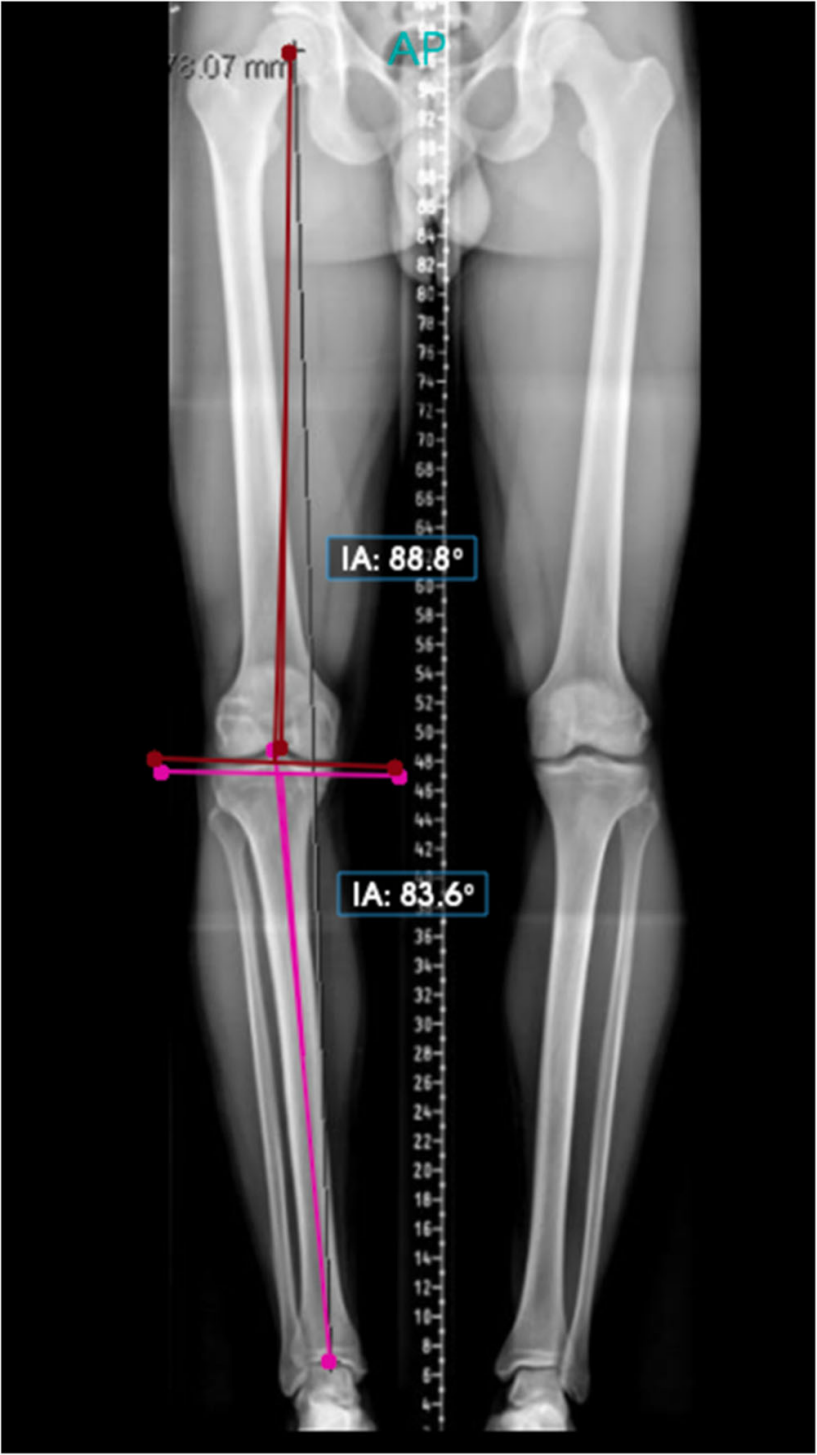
Long‐leg standing radiographs demonstrating hip–knee–ankle angle (HKA) measurement. HKA is measured as the mechanical femorotibial angle: the angle between the mechanical femoral axis (line from femoral head centre to knee centre, defined as midpoint between tibial spines) and the mechanical tibial axis (line from knee centre to talar dome centre). Values > 180° indicate valgus alignment; values < 180° indicate varus alignment. interligne angle (IA) shown by the software is the joint‐line angle.
